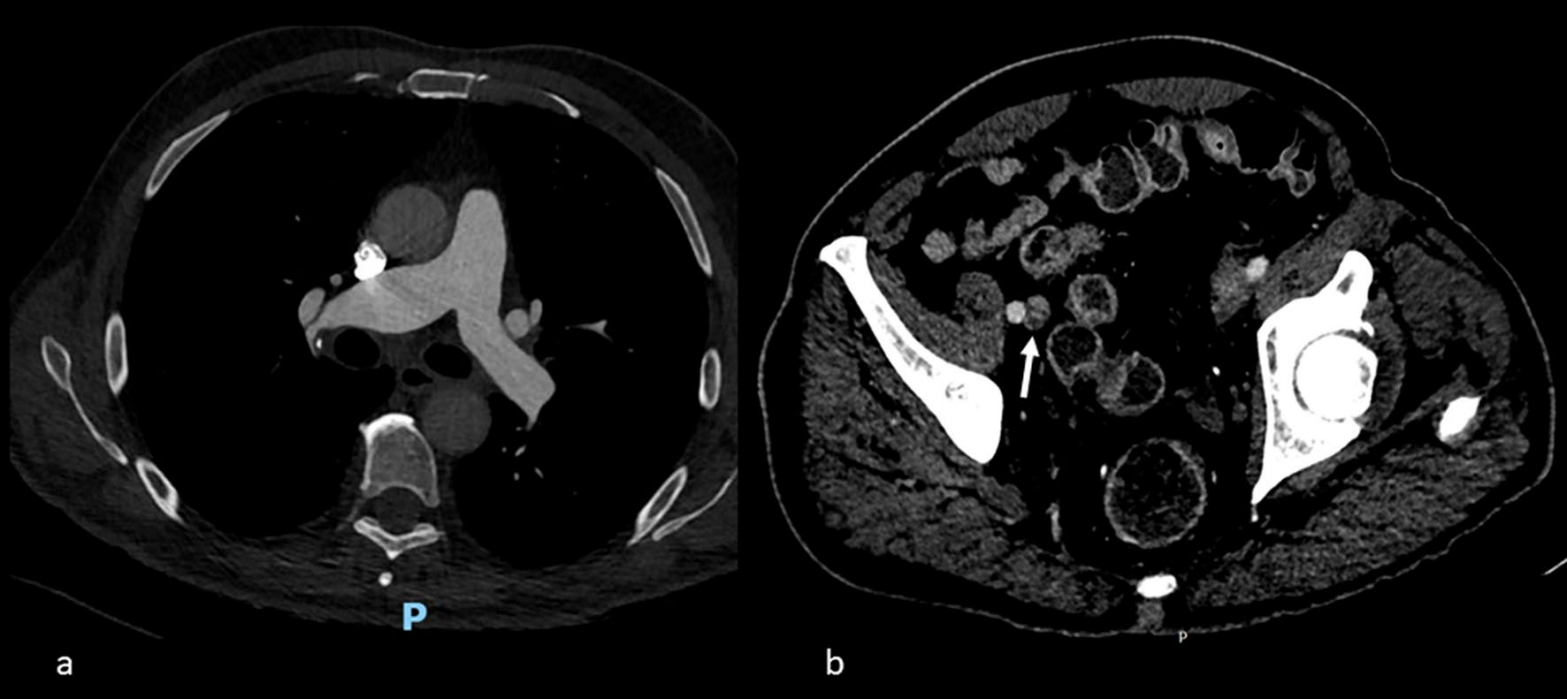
A CT scan: pulmonary artery. B CT scan: parietal thrombosis of common iliac vein (arrow)
